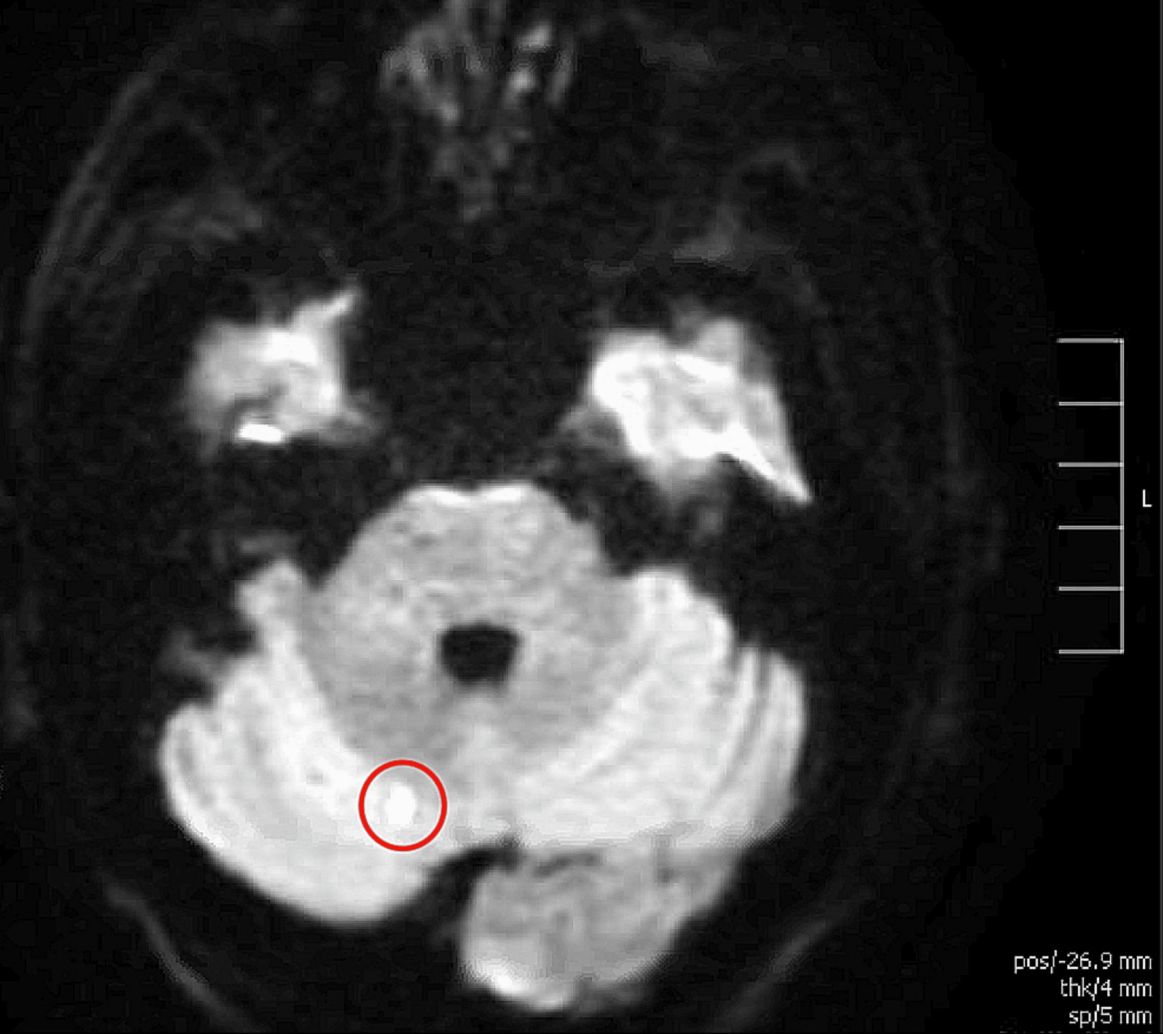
MRI of the brain showing an acute infarct in the right cerebellum
